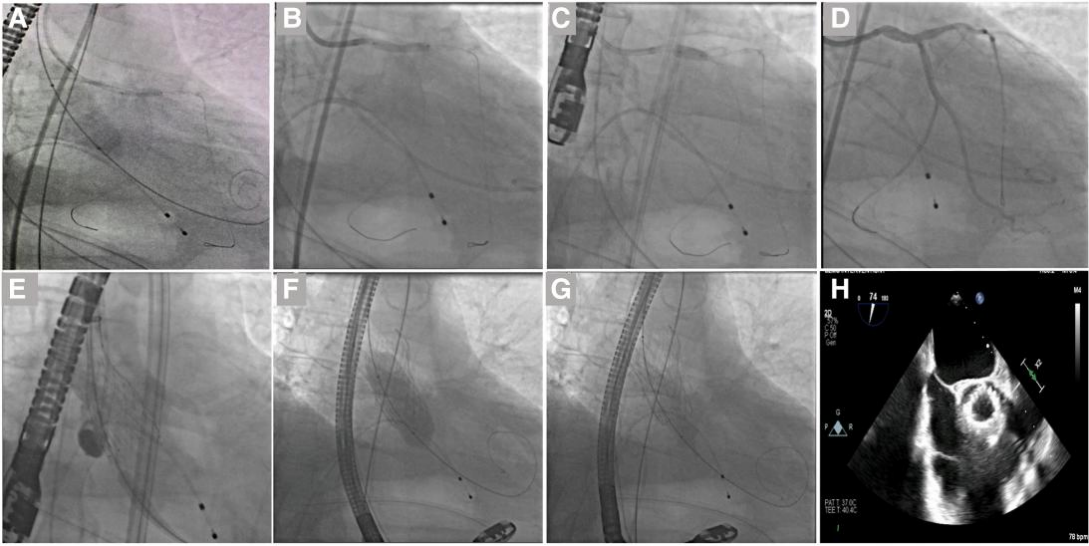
SCO consisting of BAV and LMCA balloon angioplasty during rapid pacing followed by LMCA-LAD provisional stenting and final TAVR with Evolut FX (Medtronic, Minneapolis, MN, USA). (*A*) SCO: LMCA-LAD balloon angioplasty during BAV; (*B*) LMCA stenting; (*C*) POT to the LMCA; (*D*) Post-PCI angiography; (*E*) EVOLUT FX 29 mm deployment, pigtail in non-coronary cusp; (*F*) EVOLUT FX 29 mm balloon post-dilatation; (*G*) final result; (*H*) echocardiographic shot axis view of the aortic prosthesis; BAV, balloon aortic valvuloplasty; LMCA, left main coronary artery; LAD, left anterior descending; NC, non-compliant; PCI, percutaneous coronary intervention; POT, proximal optimization technique; SCO, Simultaneous CardioFlow Optimization; TAVR, transcatheter aortic valve replacement.
